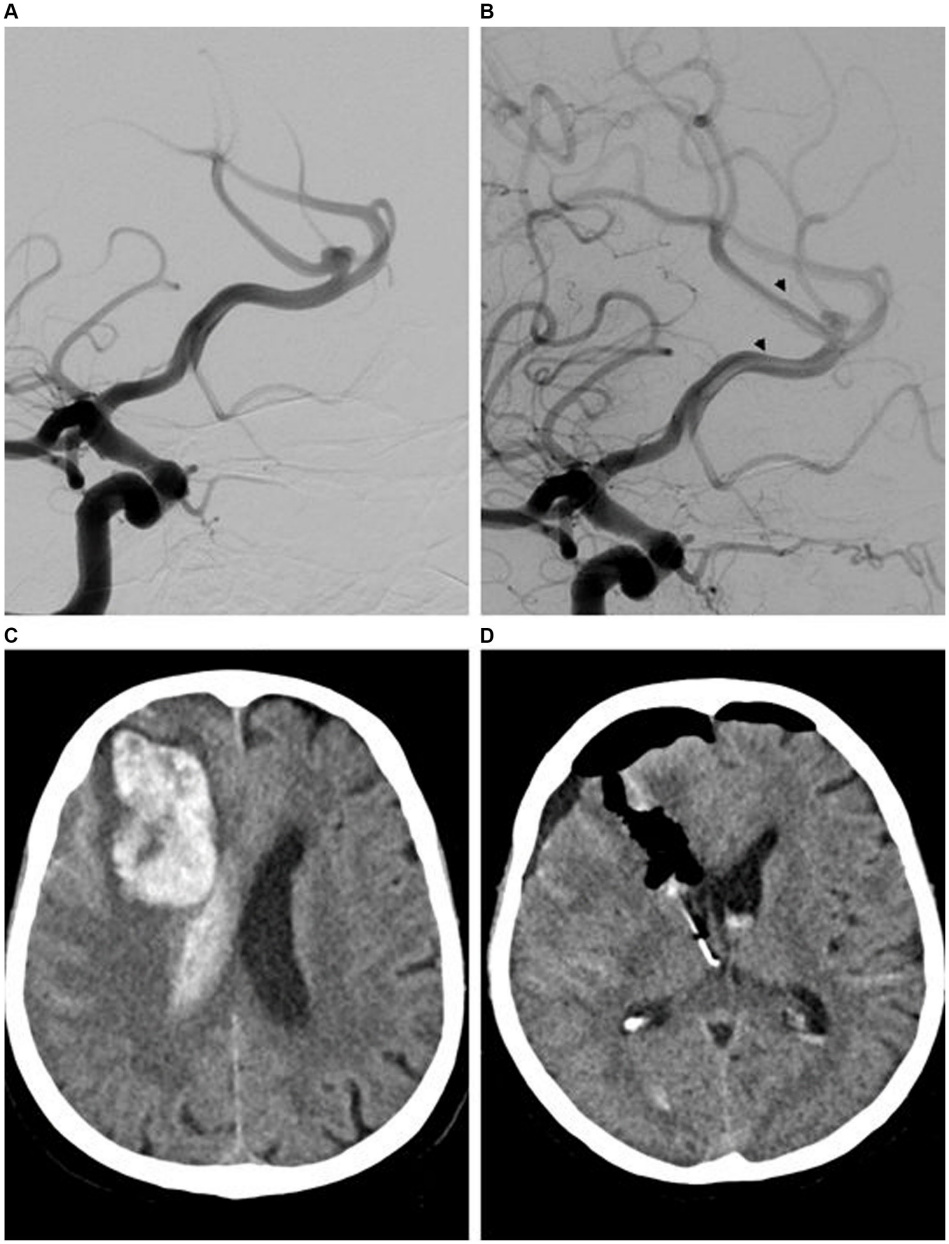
Endovascular treatment (EVT) and diagnostic imaging in a patient with a distal anterior cerebral artery aneurysm (DACAA) and periprocedural intracerebral hematoma. Digital subtraction angiography (DSA) with contrast-medium injection of the right carotid artery (ICA; lateral view 45°) revealed a DACAA at the division of the anterior cerebral artery (ACA) into the pericallosal artery (PA) and callosomarginal artery (CMA) (A). A final DSA with contrast medium injected into the right ICA highlighted cerebral circulation after implantation of the flow diverter (FD) (p64, between arrowheads) (B). The patient presented with headache and diminished vigilance on the evening of the treatment day. The subsequent computed tomography (CT) examination revealed a large parenchymal hematoma in the right frontal lobe believed to be due to hyperresponsiveness to the DAPT (ASA and ticagrelor), as confirmed by Multiplate (ADP 10 U, ASPI 11 U) and VerifyNow (P2Y12 8/255; 97%, ARU 444) analysis (C). A follow-up CT was performed after craniotomy with evacuation of the hematoma and placement of an external ventricular drain on the right frontal side (D). The patient was discharged with improved neurological status after hospitalization (mRS 2). After rehabilitation, she returned to her baseline neurologic status (mRS 0).
